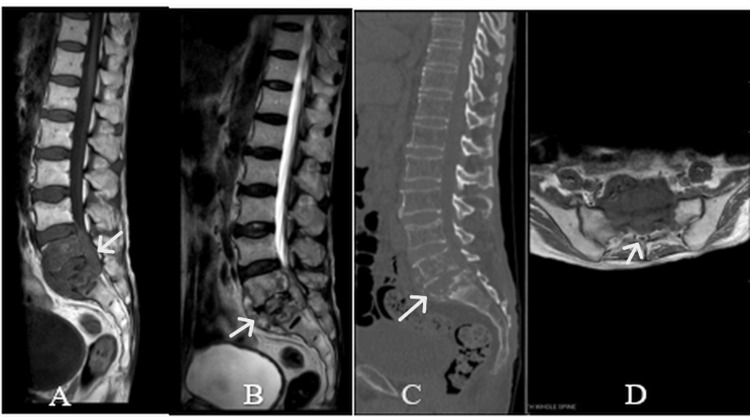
MRI of the lumbosacral spine (Case 1) (A) Sagittal T1-weighted image: hypointense marrow signal at L5 and S1 vertebral bodies (arrow indicates areas of signal loss). (B) Sagittal T2-weighted image: hyperintense inflammatory changes with L5–S1 disc involvement (arrow). (C) Post-contrast sagittal image: heterogeneous enhancement around affected vertebral endplates and intervertebral disc (arrow). (D) Axial T1-weighted image: epidural extension with canal compromise at L5–S1 (arrow). Findings are consistent with infective spondylodiscitis at the lumbosacral junction.
